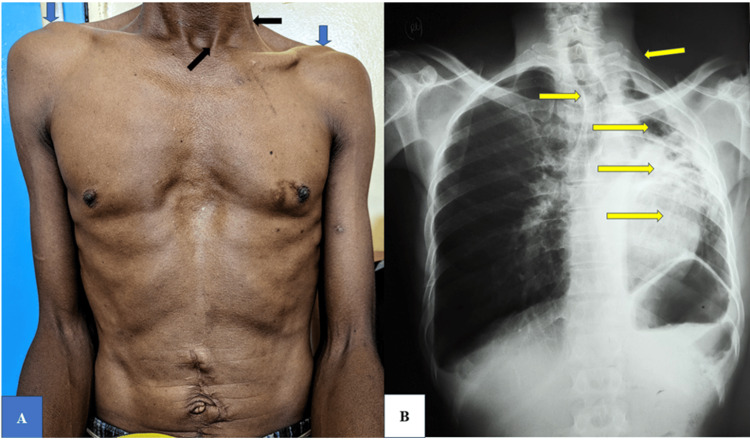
(A) Chest asymmetry observed, evidenced by the left lower acromioclavicular joint compared to the right (blue arrows). There is a positive Trail’s sign - prominence of the clavicular head of the sternocleidomastoid muscle on the left side (black arrow). Old midline surgical scar. (B) Plain radiological chest X-ray (analogue) (yellow arrows from up going downwards). Increased shadow prominence of the clavicular part of sternocleidomastoid muscle. Tracheal deviation to the left. Collapsed left upper lung. Fibrotic lung tissue. Suspected shifted mediastinum. Clear costophrenic and cardiophrenic angles. Clear right lung fields with some perihilar infiltrates.
